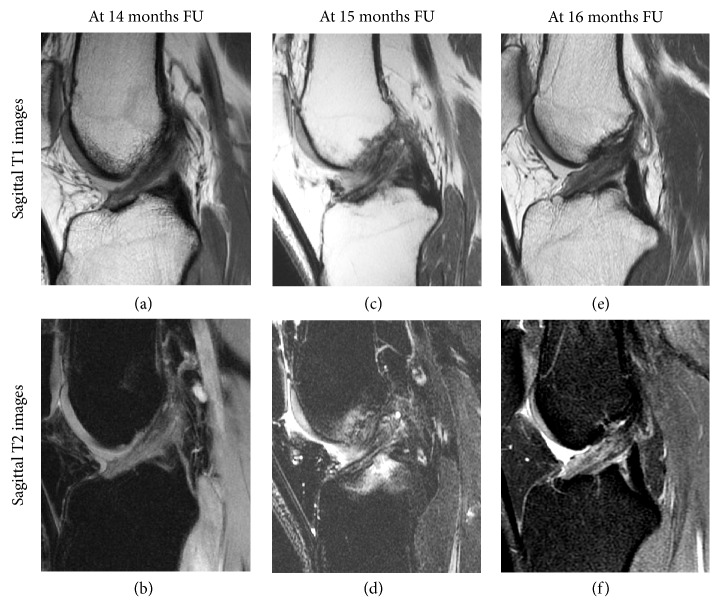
Sagittal T1 (upper row) and T2 (lower row) MR images of three patients are shown between one and two years after surgery. At this time interval, the ligament is often isointense as the ligament has more intensity than the PCL and similar intensity to the posterior muscles.
